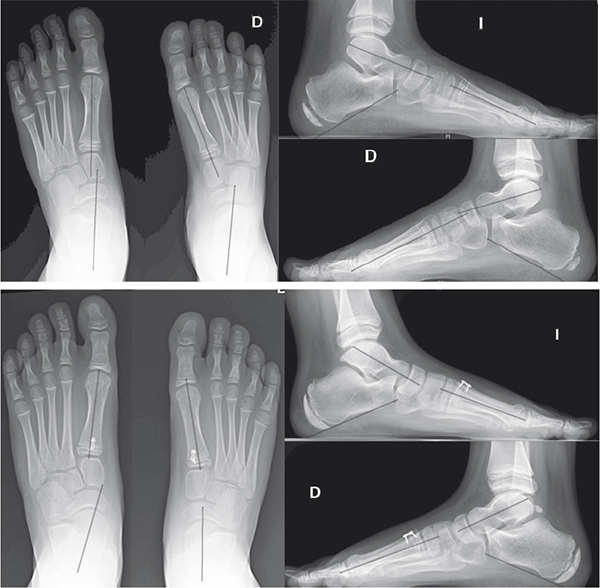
Radiographs of both feet taken preoperatively at 7 years 8 months (top), and postoperatively (bottom, same patient 18 months after surgery), to evaluate correction. On each radiograph the following angles were measured: on the AP standing view, the talus–1st metatarsal angle, and on the lateral standing view Meary’s angle and the calcaneal pitch. Note that the initial involvement was asymmetrical, with the right foot more involved than the left. By 18 months, both feet show signs of hypercorrection.
